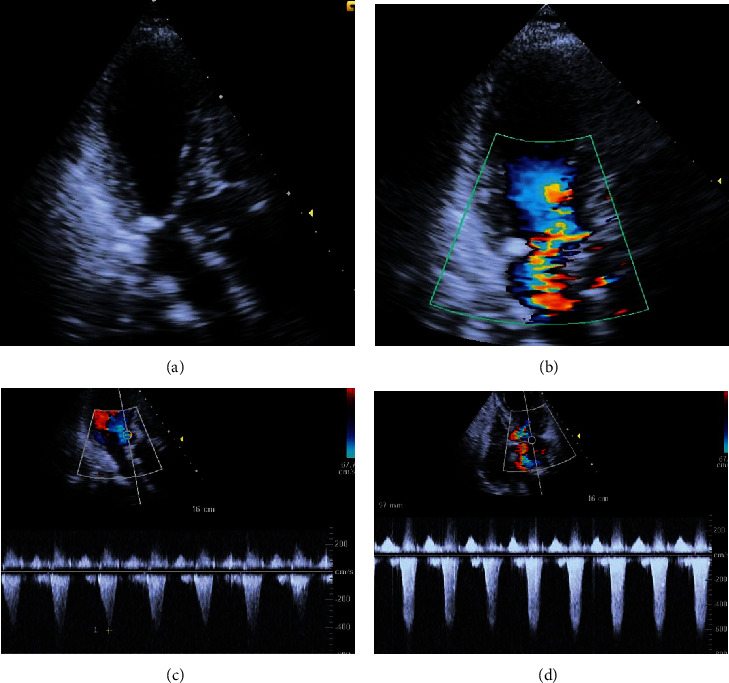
The postoperative echocardiogram performed during hemodynamic instability revealed a left ventricular outflow tract (LVOT) obstruction caused by systolic anterior movement of the mitral leaflet (SAM). (a) SAM leading to dynamic LVOT obstruction and the apical ballooning of the left ventricle. (b) A severe mitral regurgitation secondary to SAM. (c) Elevated pressure gradient across LVOT of 73 mmHg with typical dagger-shaped spectral Doppler. (d) High pressure gradient across the mitral valve in systole. The SAM and ballooning of the LV apex was seen on the apical three-chamber view (Supplement video 2).
